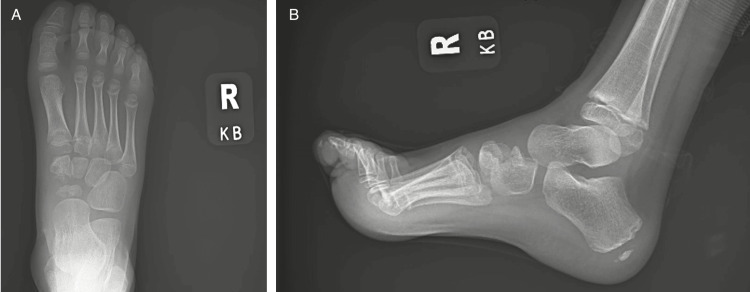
Anterior-posterior (A) and lateral (B) view radiographs were obtained eight weeks after initial diagnosis. Images demonstrate bone healing with uniform sclerosis and progressive ossification of the navicular and medial cuneiform.
